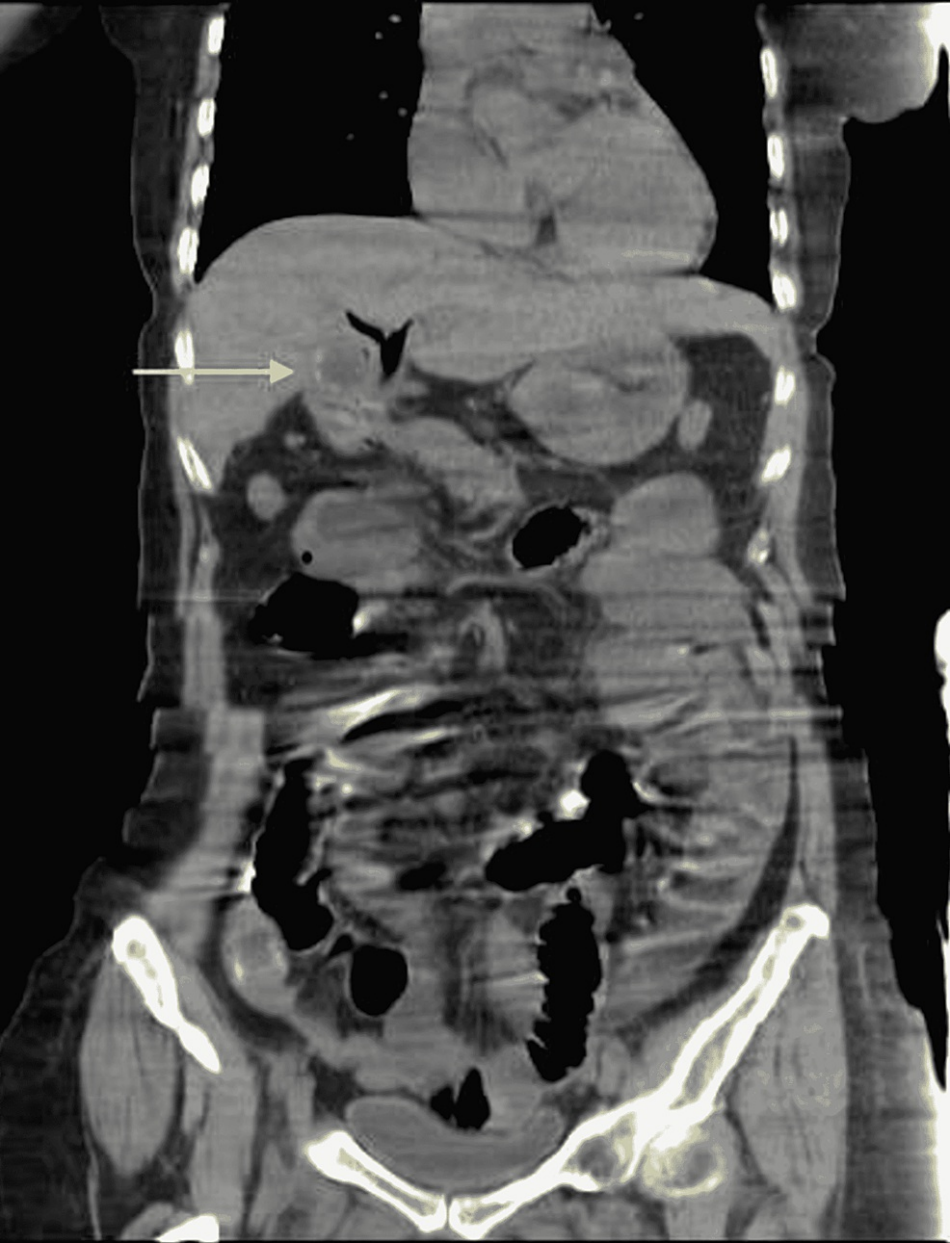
Coronal view of abdominal computed tomography Residual gallstone in the gall bladder with pneumobilia.
